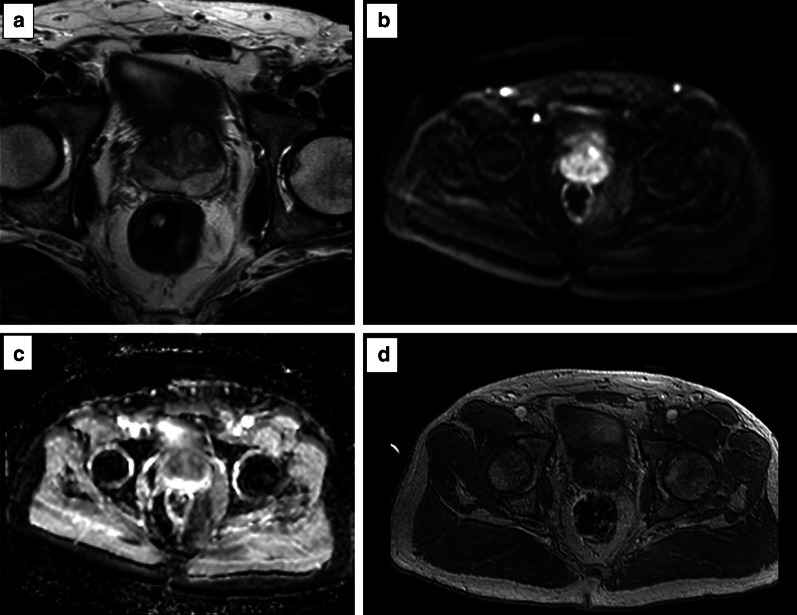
Axial T2w imaging (a), DWI with a *b* value of 150 s/mm^2^ (b), ADC map (c) and DCE acquisition (d) of a study that was given a PI-QUAL score of 1. All MR sequences are below the minimum standard of diagnostic quality as per PI-RADS v.2.1 technical recommendations. In particular, T2w imaging and DWI (a–c) show motion artefacts, no high *b* value has been acquired (b), the field of view is too large on DWI (21 × 35 cm, in b, c) and on DCE sequences (21 × 33 cm in d), and there is no fat suppression of DCE sequences (d)
